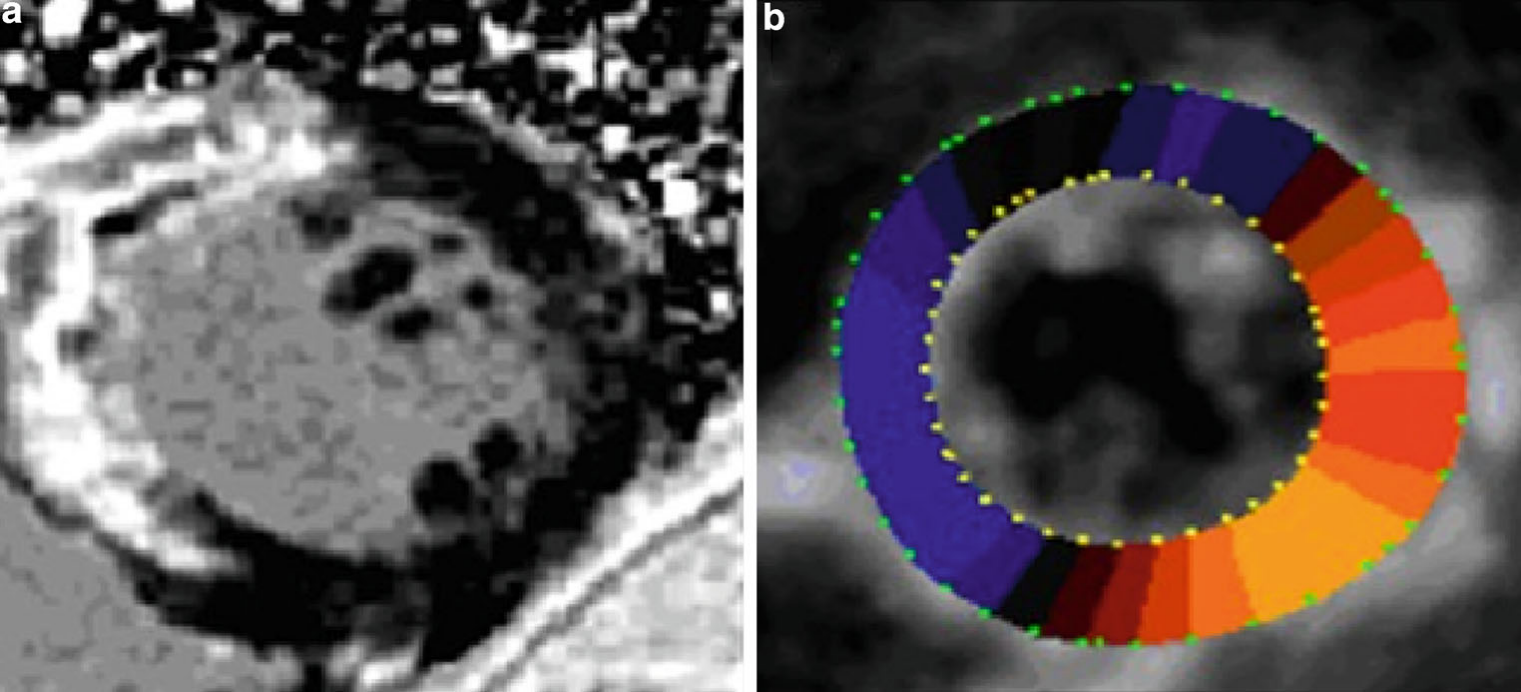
Cardiac magnetic resonance delayed contrast enhancement and three-dimensional speckle tracking echocardiography radial strain in a patient with transmural infarction. a Image of a patient with akinesia and transmural infarction of the septal and anterior walls (>50 % hyperenhancement) and (b) Colour-coded short-axis 3DSTE radial strain image at end-systole, radial strain is decreased as depicted by a blue colour overlay in a comparable region to the hyperenhancement in the CMR DCE, yet this area seems somewhat larger than the DCE one, involving the inferior wall as well. There is reddish colourisation in the other normal contracting segments with no hyperenhancement
